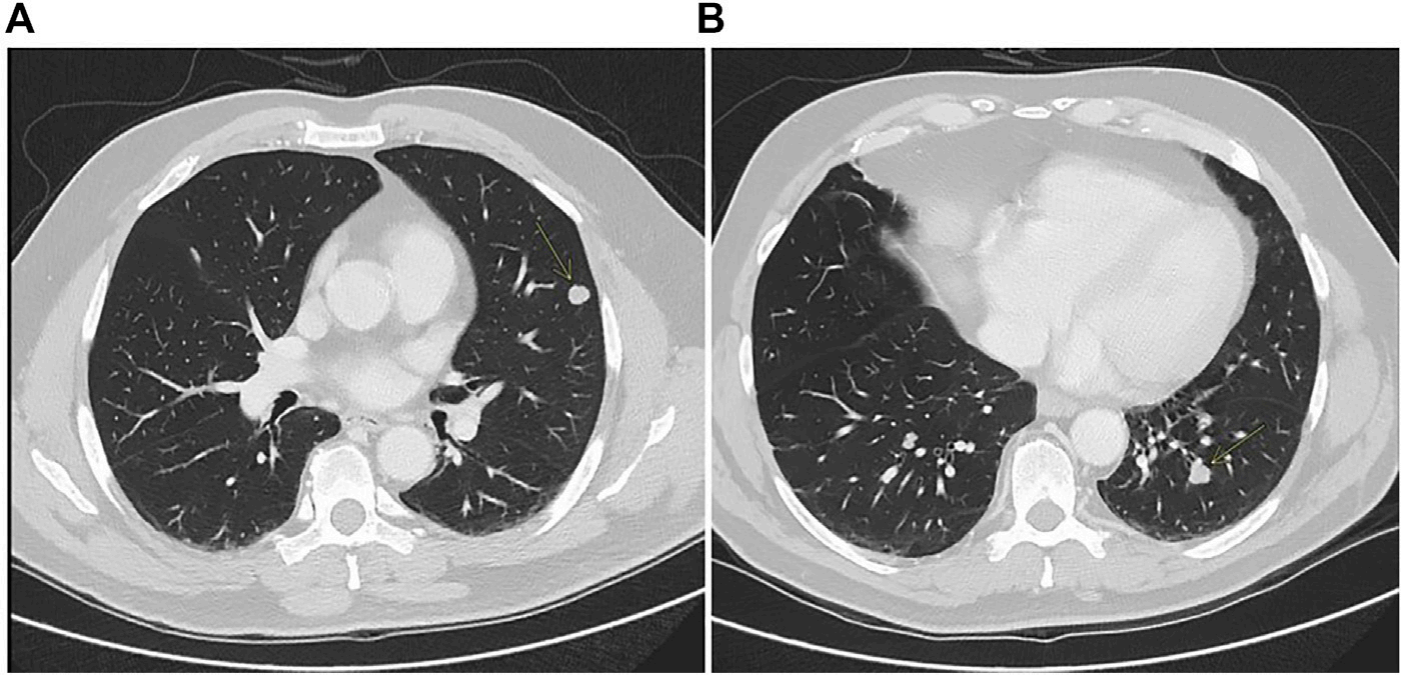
CT scan showing left lung metastases (A,B).
